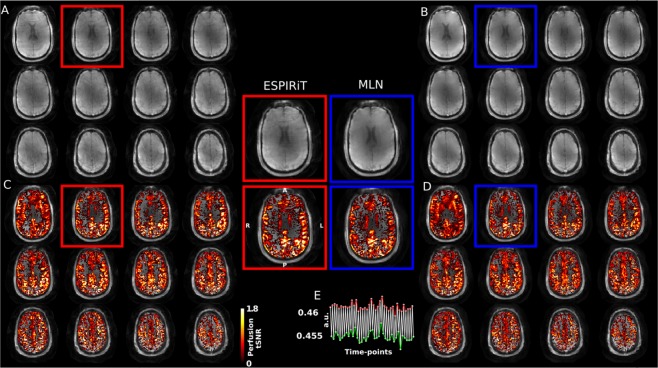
Perfusion experiment at 7 Tesla: Result of multi-slice image reconstruction from actual spiral MRI acquisitions using (A) a regularized CS-based technique (A) and the proposed method (B). Panels (C,D) show the corresponding tSNR maps. The center part shows zoomed-in versions of one selected slice. (E) Shows the ASL signal time course of the perfused region of interest where green and red colours indicate the label and control time points respectively.
